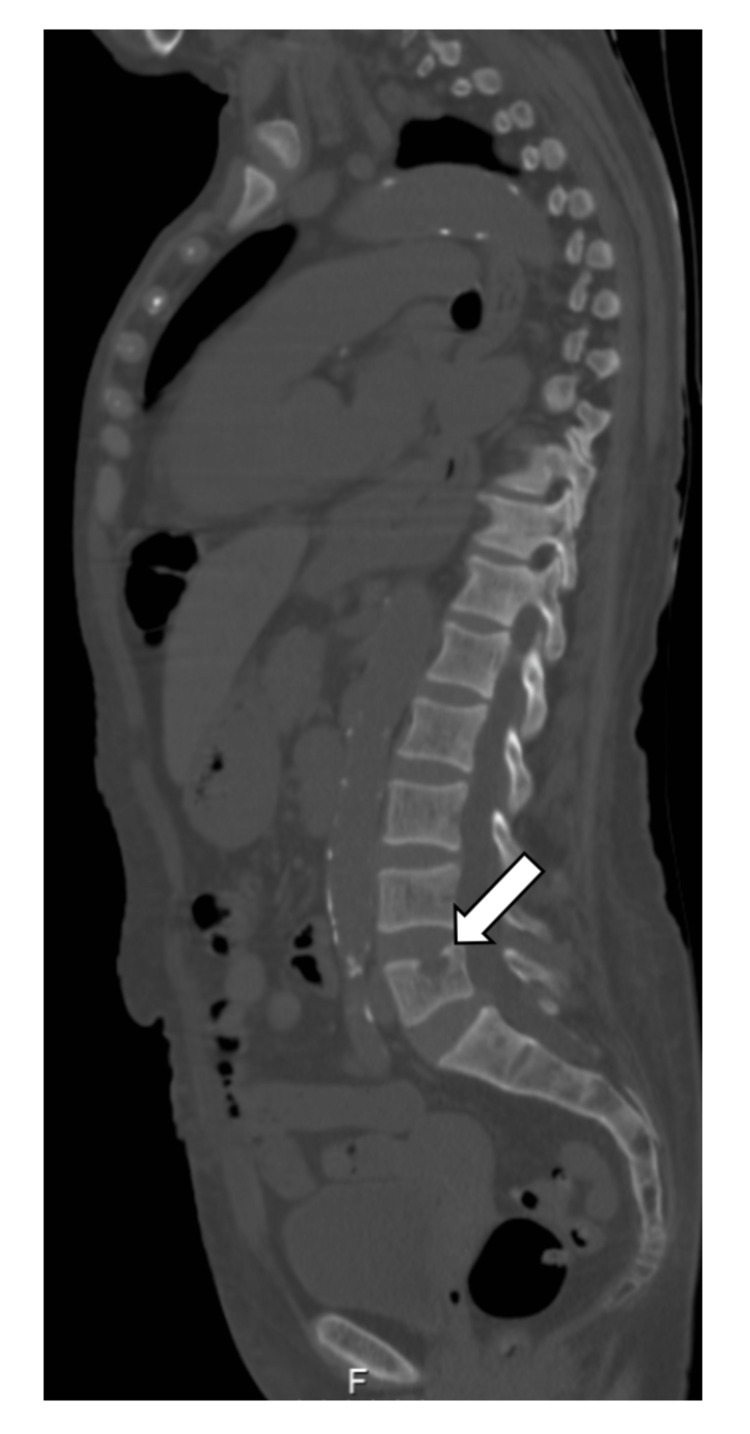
Thoracoabdominopelvic CT scan. Sagittal view of thoracoabdominopelvic CT scan showing a well-defined lytic image on the upper vertebral platform of L5 (arrow) with pericentimetric dimensions.
